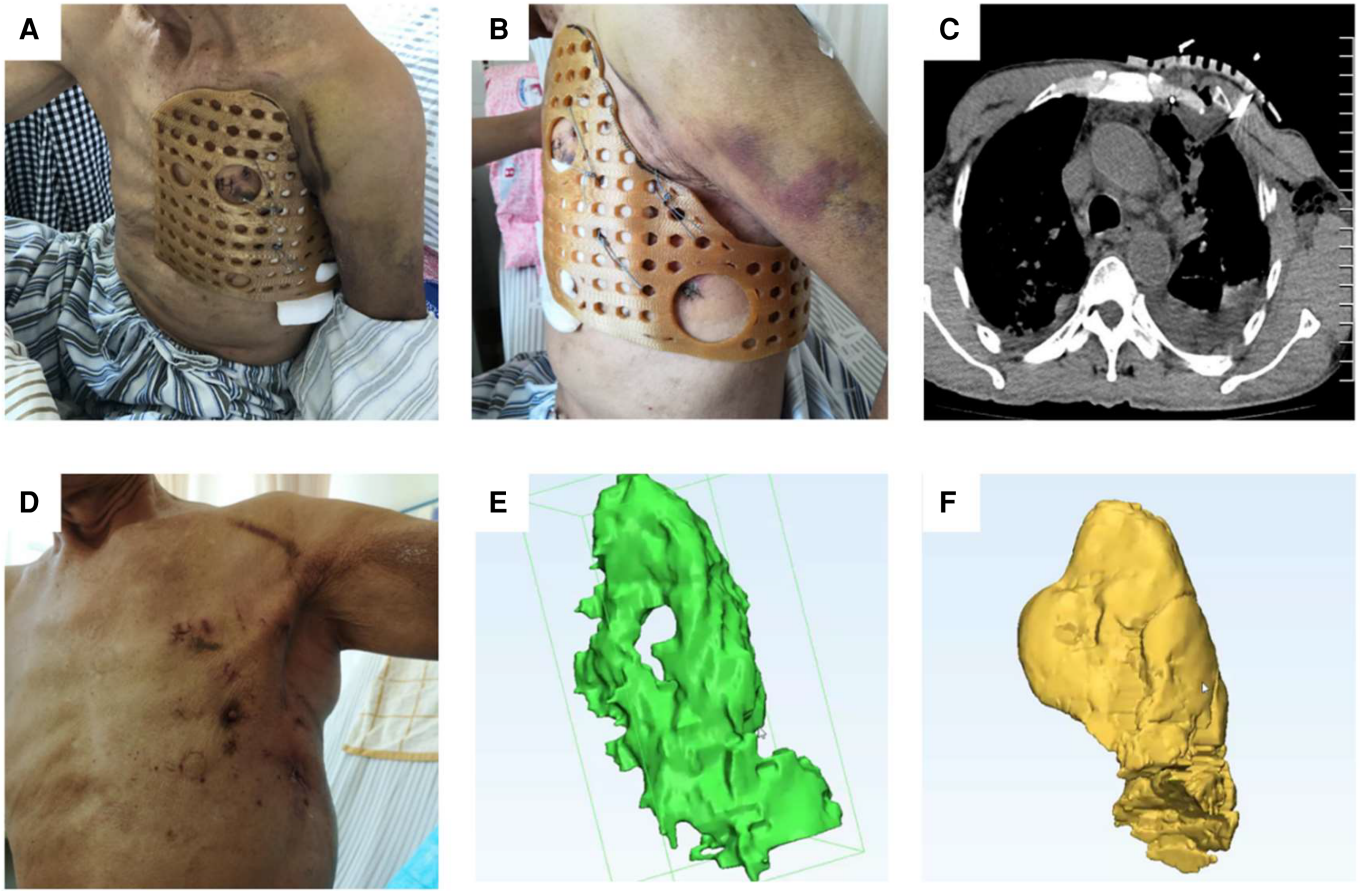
After the operation, the 3D-printed guide plate was firmly and closely fitted to the chest wall from different visual fields (A,B). Reexamination of the chest CT showed that the broken end of the rib fracture was reduced and the shape of the chest was reconstructed (C,D). Modeling was performed using MIMICS software, and the green area represents the preoperative thoracic volume (E) The yellow area represents the postoperative thoracic volume, which increased significantly compared to the preoperative one (F).
